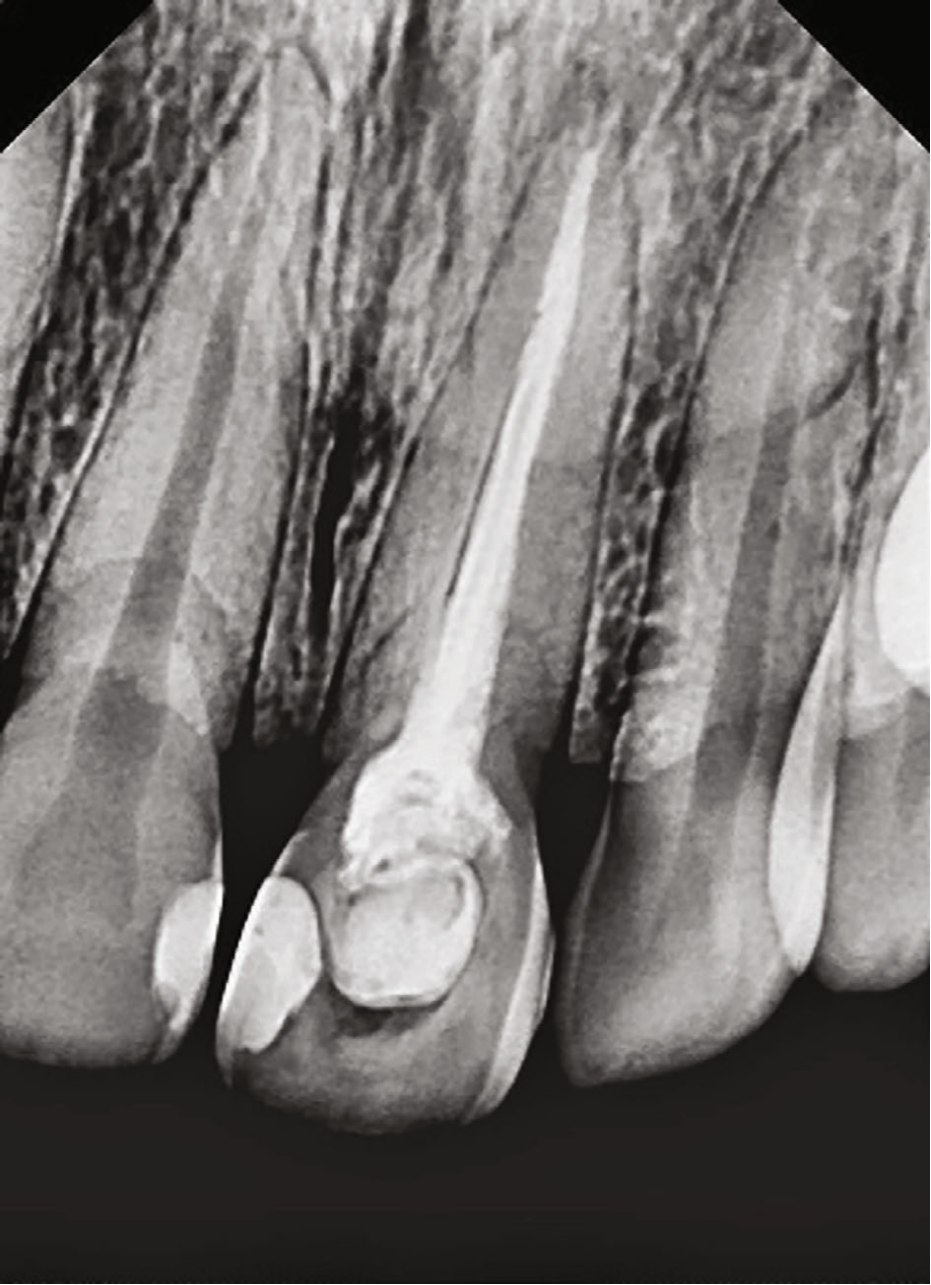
Initial radiograph showing external root resorption on Tooth 21.
